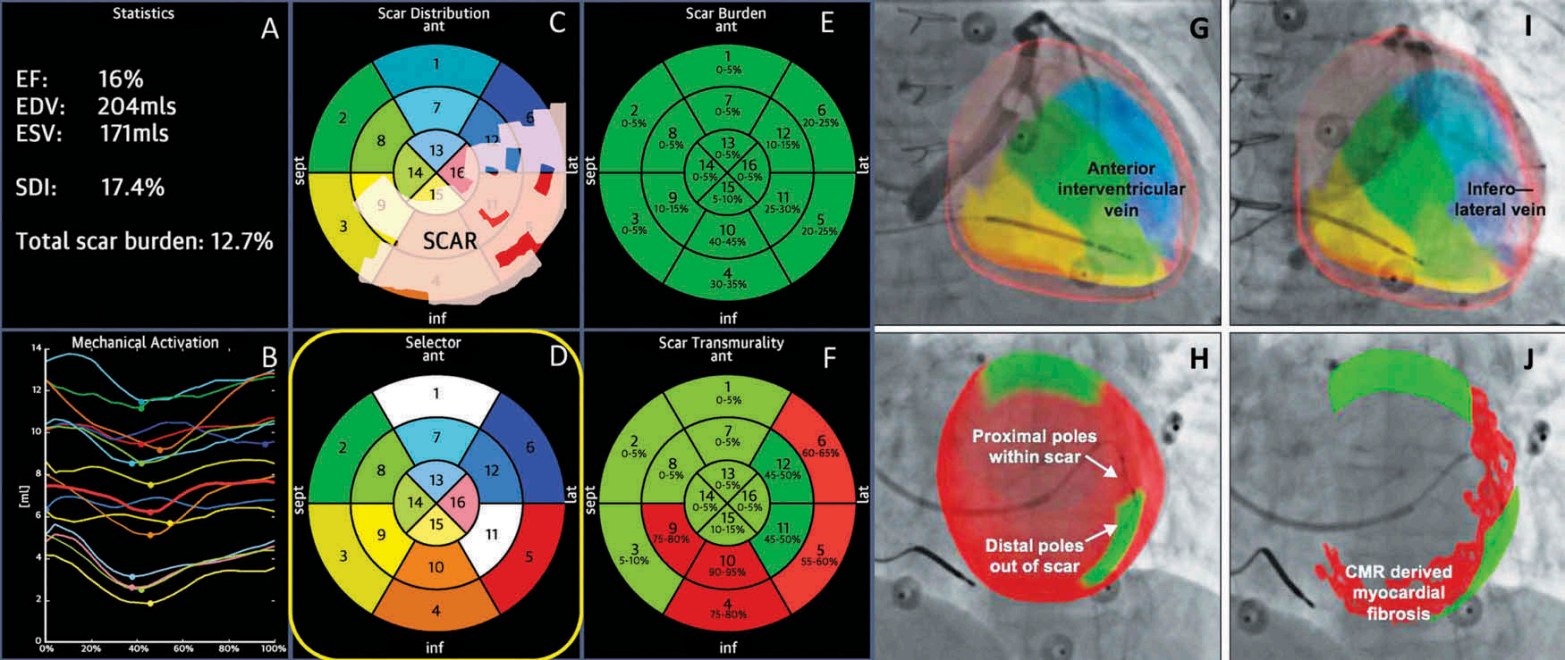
(A) (Top left) Anteroposterior venogram with overlay of CMR-derived epicardial/endocardial shell with 16-segment American Heart Association model showing an anterior interventricular vein. The 3D CMR-derived shell has the same colors as displayed in the guidance platform as shown in Figures 2 and 3. Infero-septal, antero-septal, and anterior segments are colored in yellow, green, and blue, respectively. (Top right) left anterior oblique (LAO) 20 venogram with automated rotation and alignment of the 16-segment model with the x-ray. Inferolateral veins are demonstrated. (Bottom left) LAO 40 projection. The positioning of a quadripolar left ventricular lead into a preselected target segment (green). (Bottom right) LAO 40 projection, alternate view with CMR-derived scar distribution (red). Attempted positioning and pacing using left ventricular poles out of regions of scar. Reproduced with permission from Elsevier due to the creative commons license. (B) This display screen is seen following the processing of the CMR data set and is mimicked on the large screen in the catheter laboratory. Total scar burden calculated as a mean of all myocardial segments. (Top middle) Scar distribution denoted in grey upon an American Heart Association 16-segment model. (Top right) Scar burden (% scar per myocardial segment volume), displayed in 5% ranges. (Bottom right) Scar transmurality demonstrating the mean transmurality from endocardium to epicardium. Those segments >50% transmural myocardial fibrosis are also denoted in red. (Bottom left) Mechanical activation curves for the 16 segments, corresponding to the colors shown in the middle panels. Endocardial tracking of the left ventricle provides absolute changes in the volume per segment (ml, y axis) over the cardiac cycle (0% end diastole, 30% to 50% end systole, 100% end diastole). Because these are absolute volume changes, the apical segments are always at the bottom because they have a smaller start and end volume. When the user hovers over a segment in the top middle panel, the associated volume time curve appears in bold; in this case, the target posterolateral segment is shown. (Bottom middle) Target selection panel. Upon reviewing the scar location, burden, transmurality, and mechanical activation curves, target segments are chosen (seen here in white; basal anterior, mid-posterolateral). EDV 1⁄4 end-diastolic volume; EF 1⁄4 ejection fraction; ESV 1⁄4 end-systolic volume; SDI 1⁄4 systolic dyssynchrony using endocardial tracking of CMR cine images in short and long axis. Reproduced with permission from Elsevier due to the creative commons license. Full color available online.
